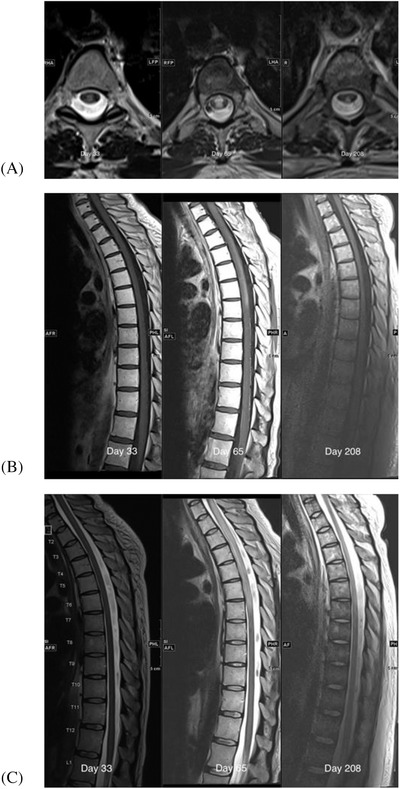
MRI images D+33, D+65 and D+208: (A) axial T2 weighted, (B) sagittal T1 weighted post‐gadolinium, and (C) sagittal T2 weighted images
